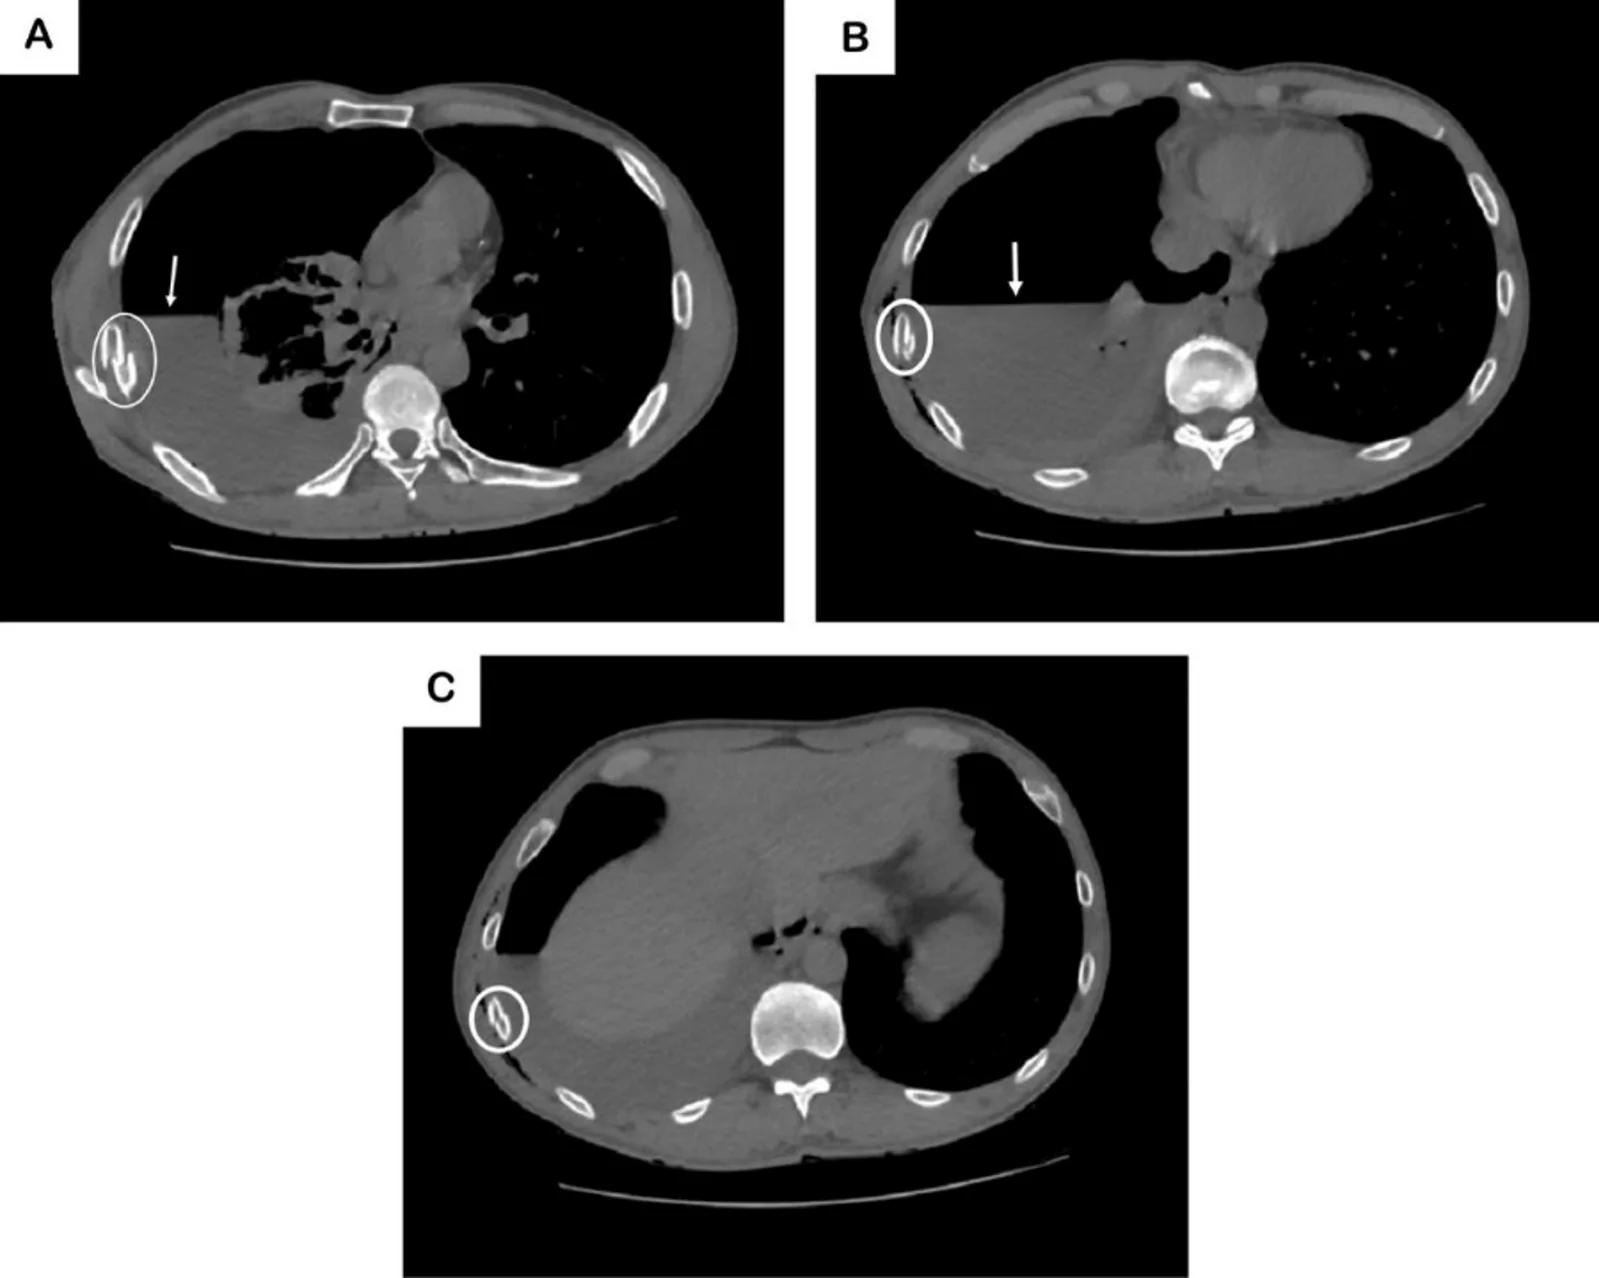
Non-contrast axial chest computed tomography (CT) scans (bone window), taken on day one of admission. (A) Chest CT demonstrates a lateral fracture of the right seventh rib (white circle), accompanied by a right-sided hydropneumothorax with a prominent flat air-fluid level (white arrow). (B) Chest CT demonstrates a displaced lateral fracture of the right eighth rib (white circle), accompanied by a right-sided hydropneumothorax with a prominent flat air-fluid level (white arrow). (C) Chest CT demonstrates a posterior fracture of the right ninth rib (white circle).
